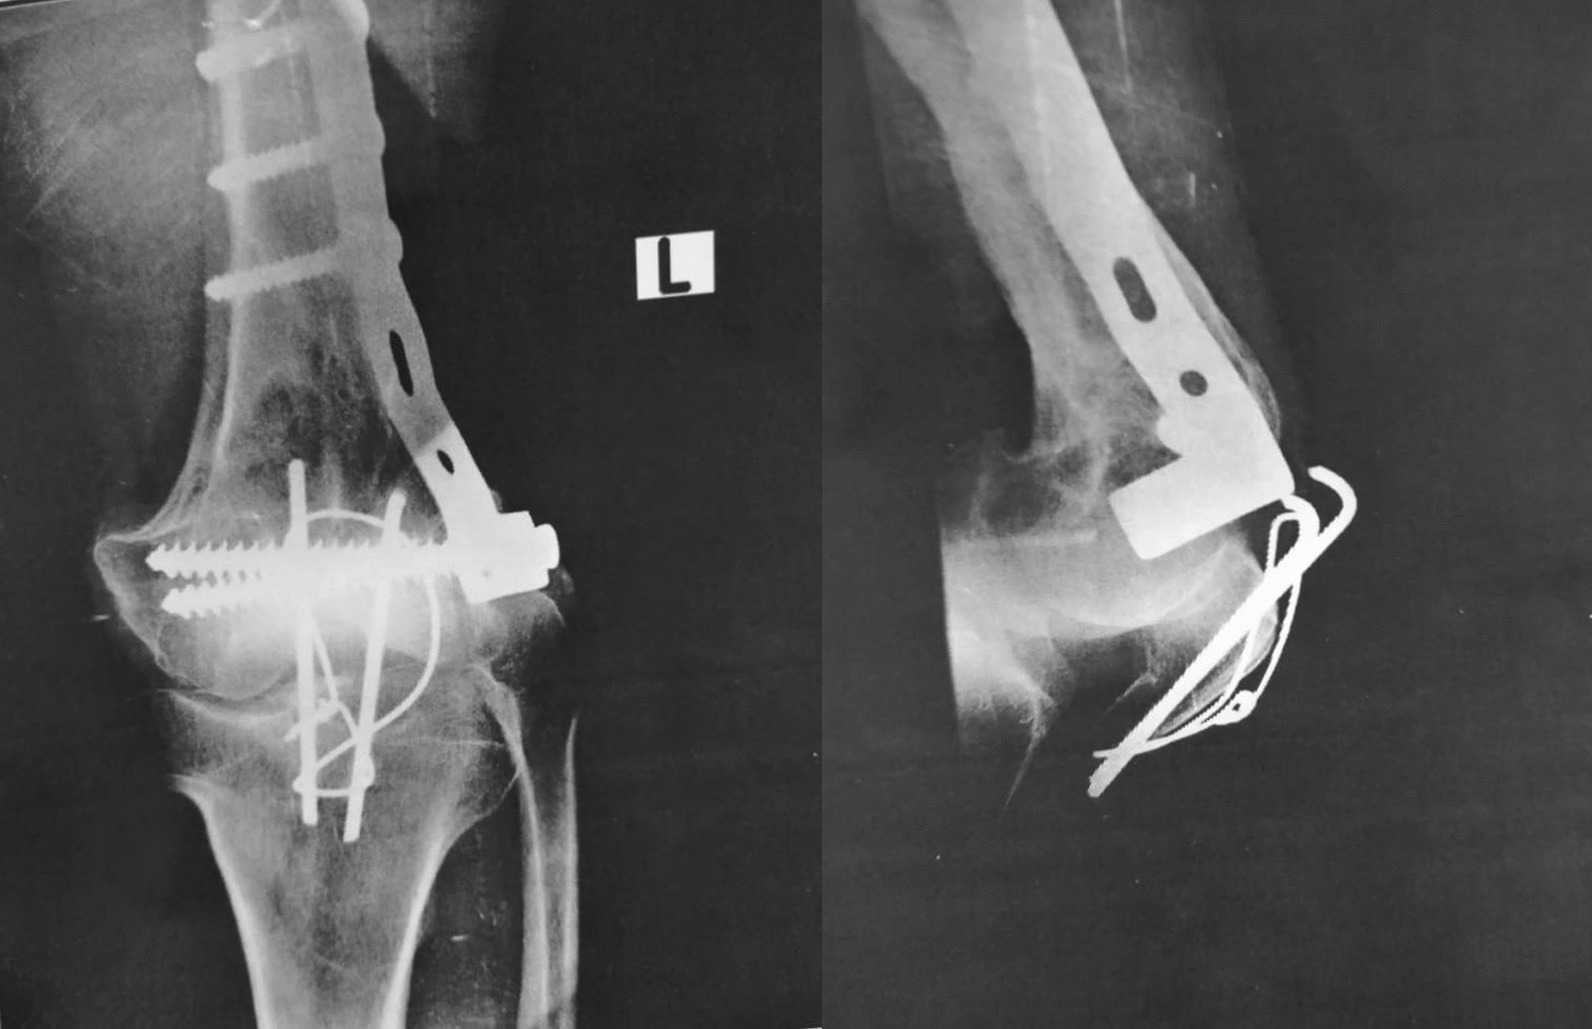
3 years post operative X-ray images showing the consolidation of the fractures
